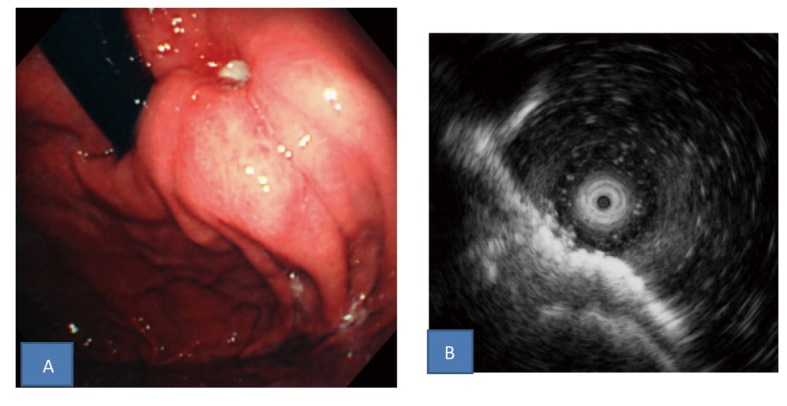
A: Upper gastrointestinal endoscopic findings after Hp eradication therapy followed by 8 weeks of PPI administration. The ulcer slightly shrunk without scarring and the surrounding mucosa was markedly elevated like a submucosal tumor. B: Endoscopic ultrasonography findings. Thickening of the submucosal and muscular layers around the ulcer was found.
